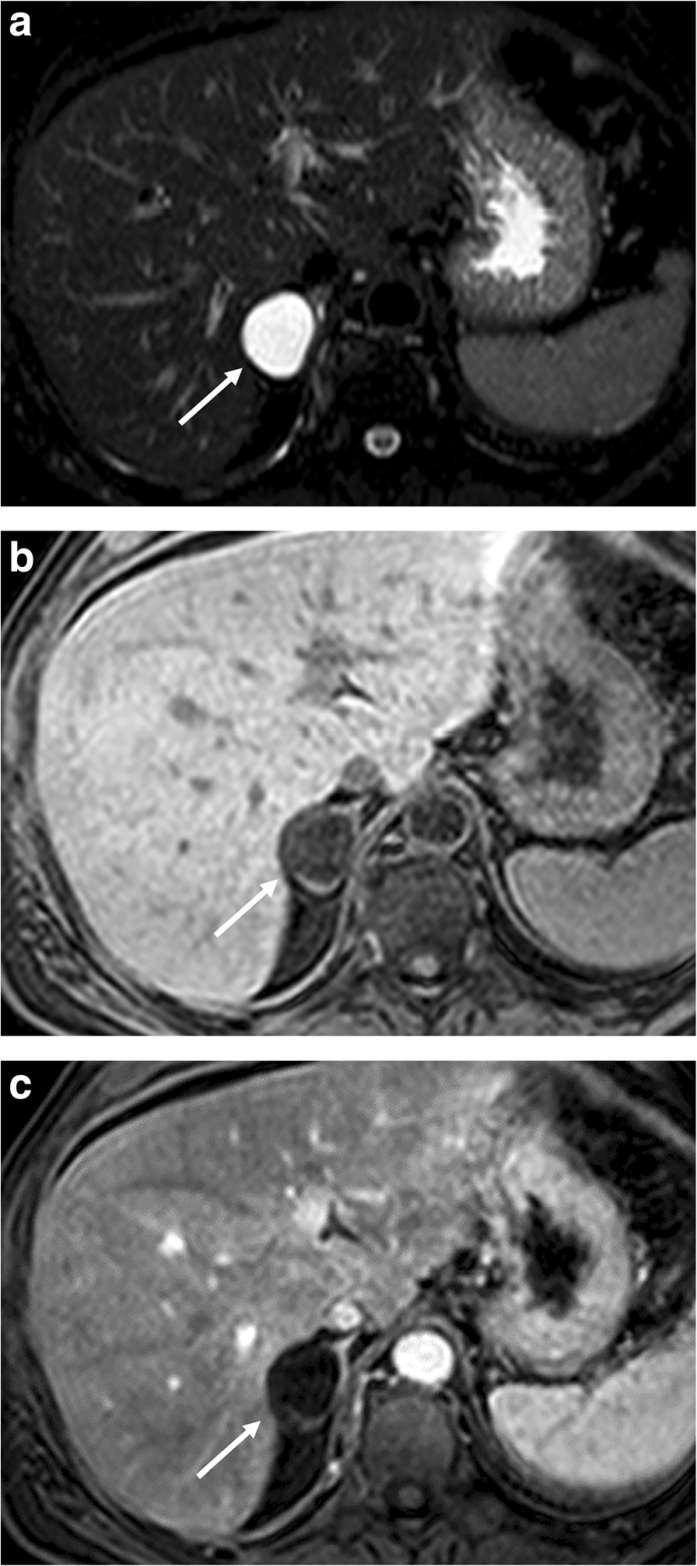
Right adrenal cyst in a 50-year-old woman. Axial T2-weighted fat-saturated fast spin echo MR image (a), three-dimensional fat-suppressed T1-weighted gradient recalled echo images before (b) and after paramagnetic contrast administration (c) show a right adrenal cystic lesion (arrow) with low T1 signal intensity, high T2 signal intensity, no contrast enhancement and no septations, blood products, soft-tissue components, or calcifications
